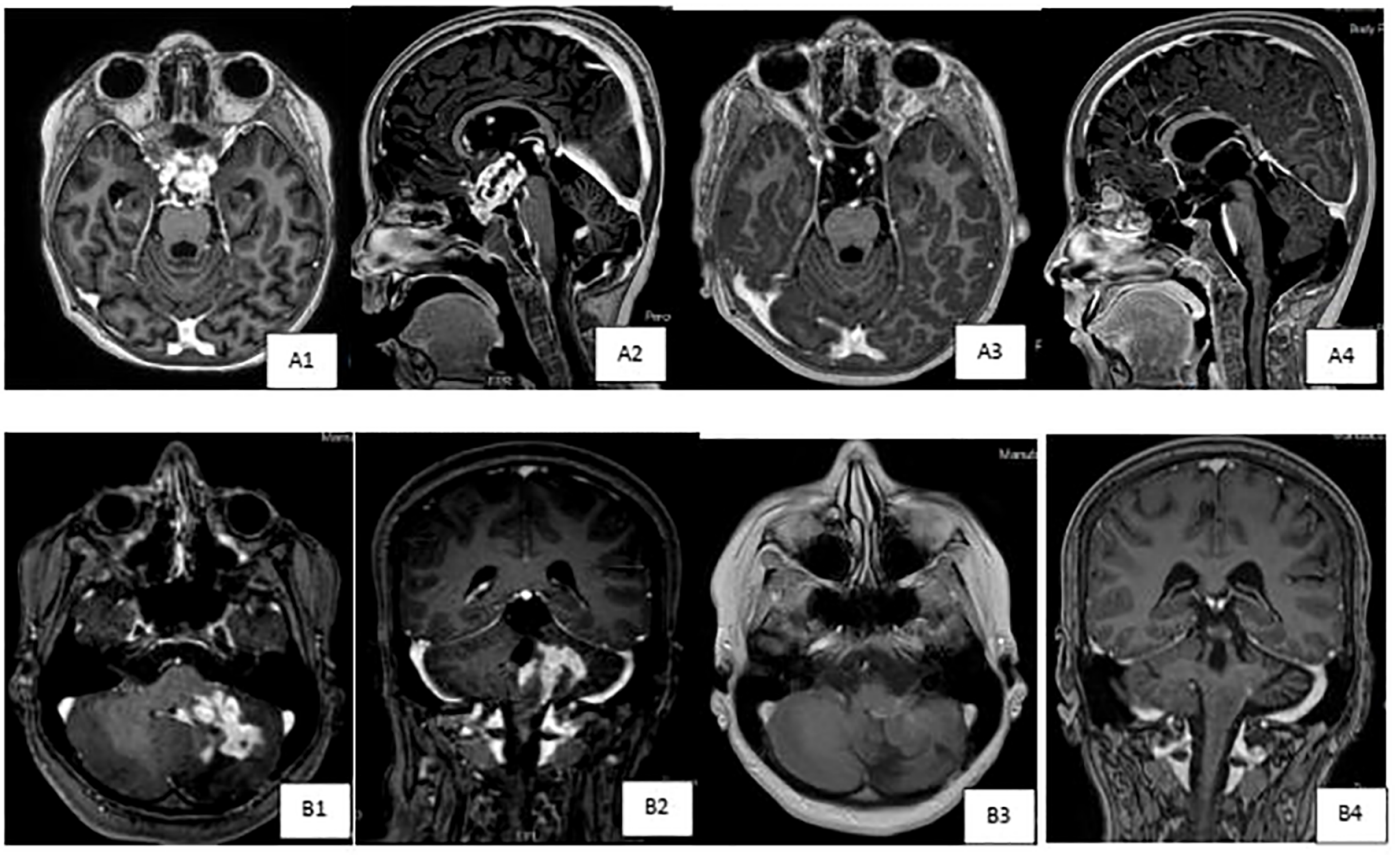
Brain MRI scans demonstrating tumor response to targeted therapy in two patients with low grade gliomas. (A) Axialand sagittal T1-weighted post IV contrast brain images of patient # 14 with diffuse leptomeningeal glioneuronal tumor (DLGNT),demonstrating pretreatment (A1& A2) large contrast enhancing mass in the suprasellar cistern,inseparable from the optic chiasm,extending to the floor of the third ventricle. Subependymal,intraventricular enhancing nodules are also noted, seen onlower row images. Marked interval tumor response {A3 & A4) with resolution of cortrast enhancement with almost resolution of previously seen subependymal enhancing nodules,currently much smaller and non­enhancing, as seen in upper row images. (B) Axialand coronal T1-weighted post IV contrast brain images of patient # 13 with cervico-medullary gangiloglioma,demonstrating pretreatment (B1 & B2) heterogeneous contrast enhancingmass in the left cerebellar hemisphere,with the involvement of the brain stem,particularly l eft hemi medulla,and leptomeningealenhancement extending to the left foramen of Luschka.Marked interval tumor response (B3 & B4) in the tumoral component within theleft cerebellar hemisphere, with almost resolution of mass like contrast enhancement,development of leukomalacia, improvement in the expansion of the left hemi medulla and contrast enhancement.
